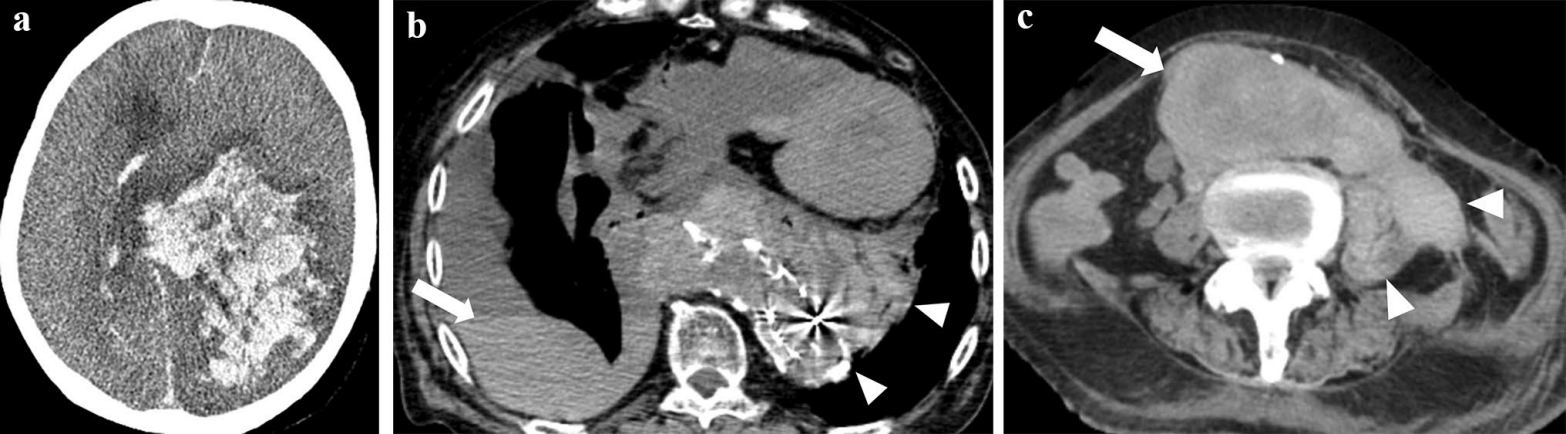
Examples of diagnosable fatal pathology on postmortem CT. a cerebral hemorrhage in the left parietal lobe with subfalcine herniation. b right hemothorax (arrow) due to ruptured thoracic aortic aneurysm after endovascular aortic repair (arrowheads). c ruptured abdominal aortic aneurysm (arrow) and massive retroperitoneal hemorrhage (arrowheads)
